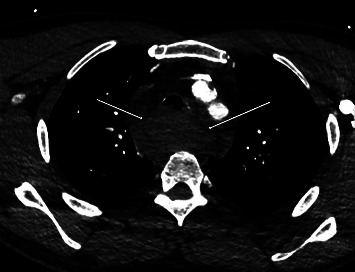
CT image demonstrating hyperdense collection along the anterior margin of the C7 vertebral body.
